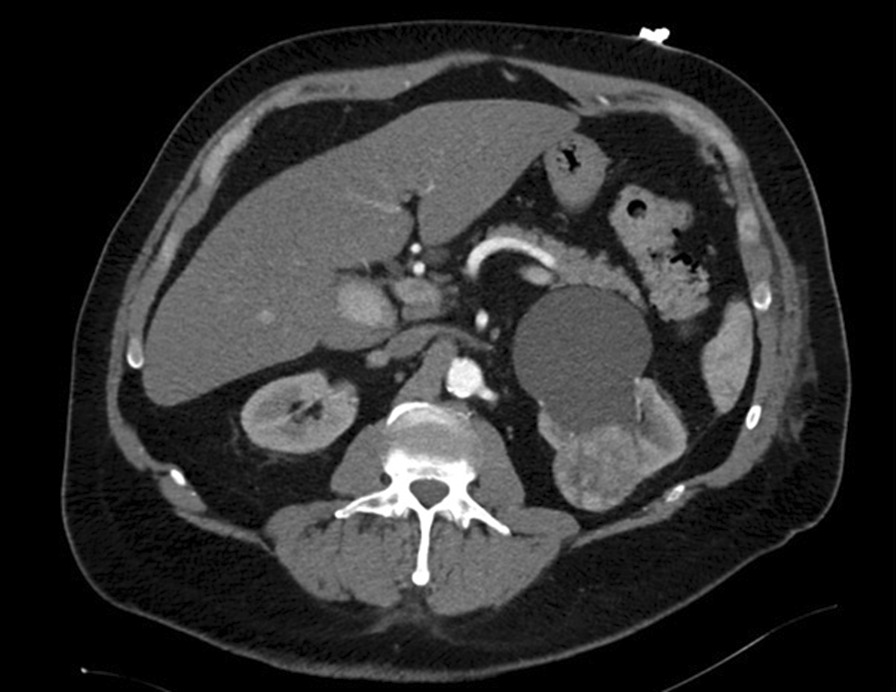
Abdominal CT scan showing a renal tumor of the left side with adjacent renal cyst
